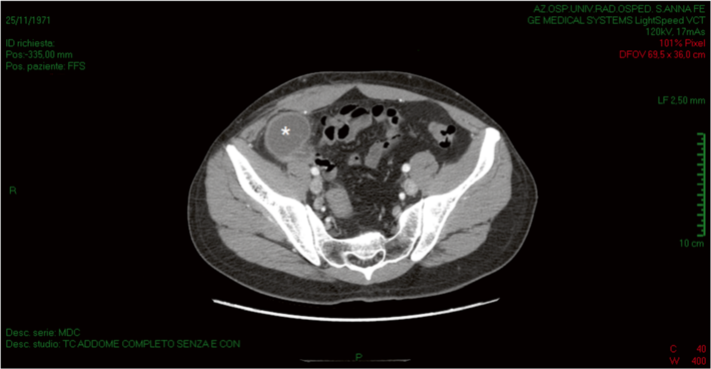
Abdominal computed tomography. Cystic mass (*asterisk*) between the cecum and the right parietocolic recess
